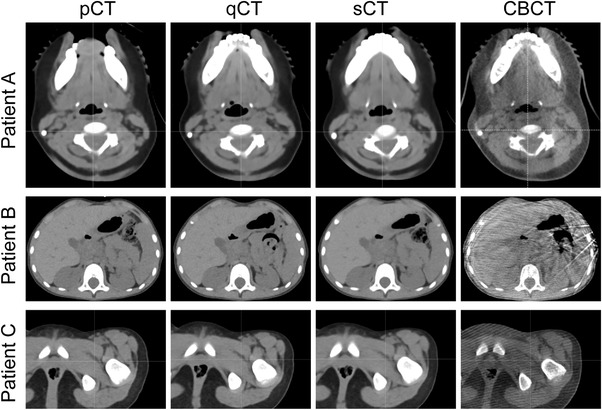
Example plan CT (pCT), quality assurance CT (qCT), synthetic CT (sCT), and cone‐beam CT from three example patients
